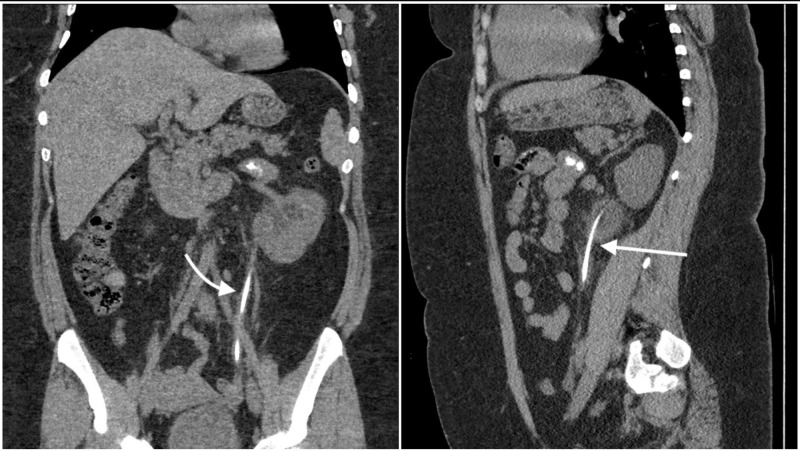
Coronal (left) and sagittal (right) view of the patient's abdominal CT showing an encrusted ureteral stent
